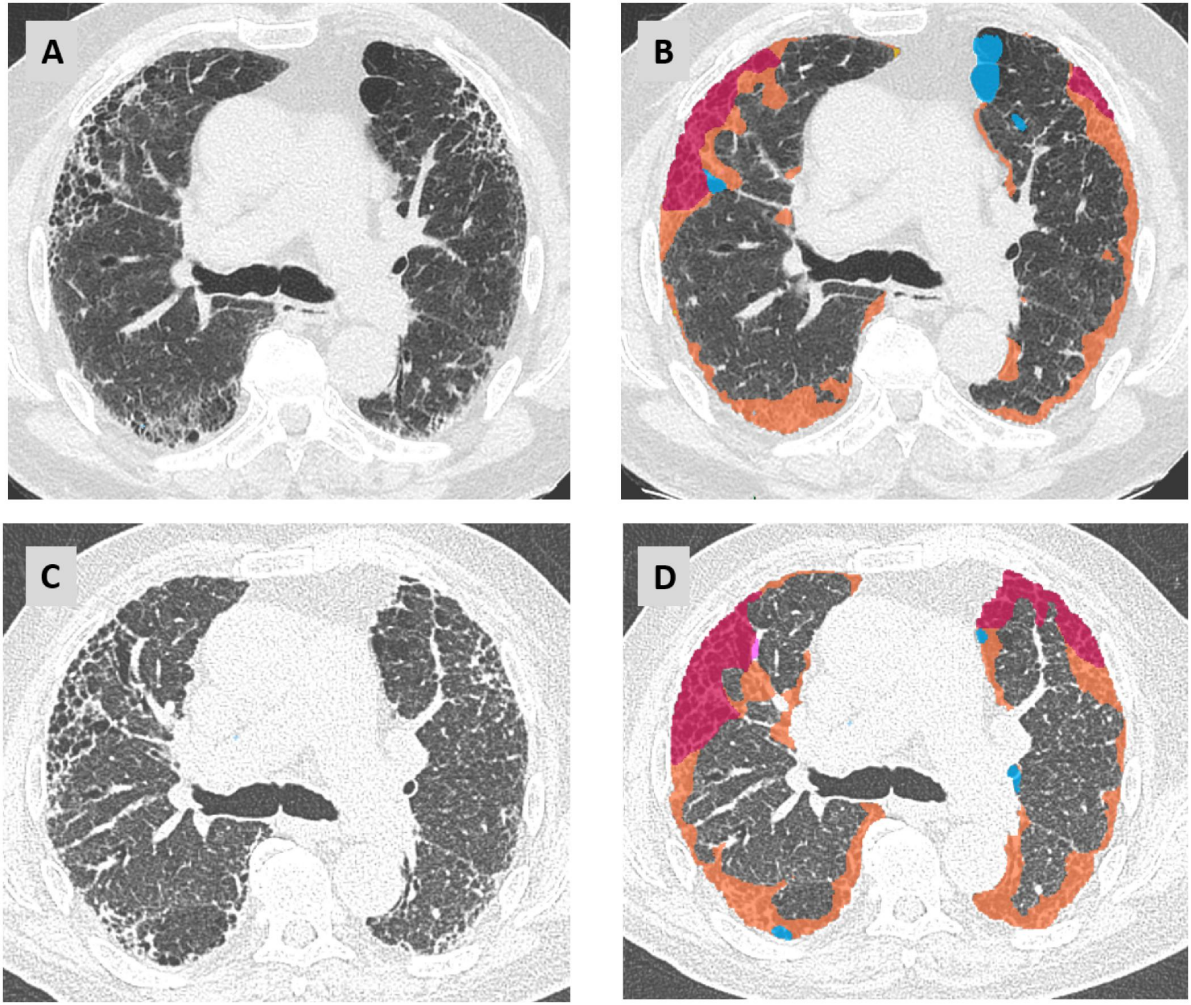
Example of a commercially available AI-based QCT algorithm (AVIEW Lung Texture, Coreline Soft, Seoul, Korea). Serial CT scans from a patient with RA-ILD exhibiting a usual interstitial pneumonia (UIP) pattern. Axial images at the level of the carina from 2019 are shown as native (A) and with QCT overlay (B), highlighting fibrotic changes with honeycombing (pink) and reticulations (orange). Paraseptal emphysema is marked in blue. Follow-up scans from 2022 (C and D) demonstrate progression of fibrosis with a slight increase in honeycombing in the right upper lobe.
